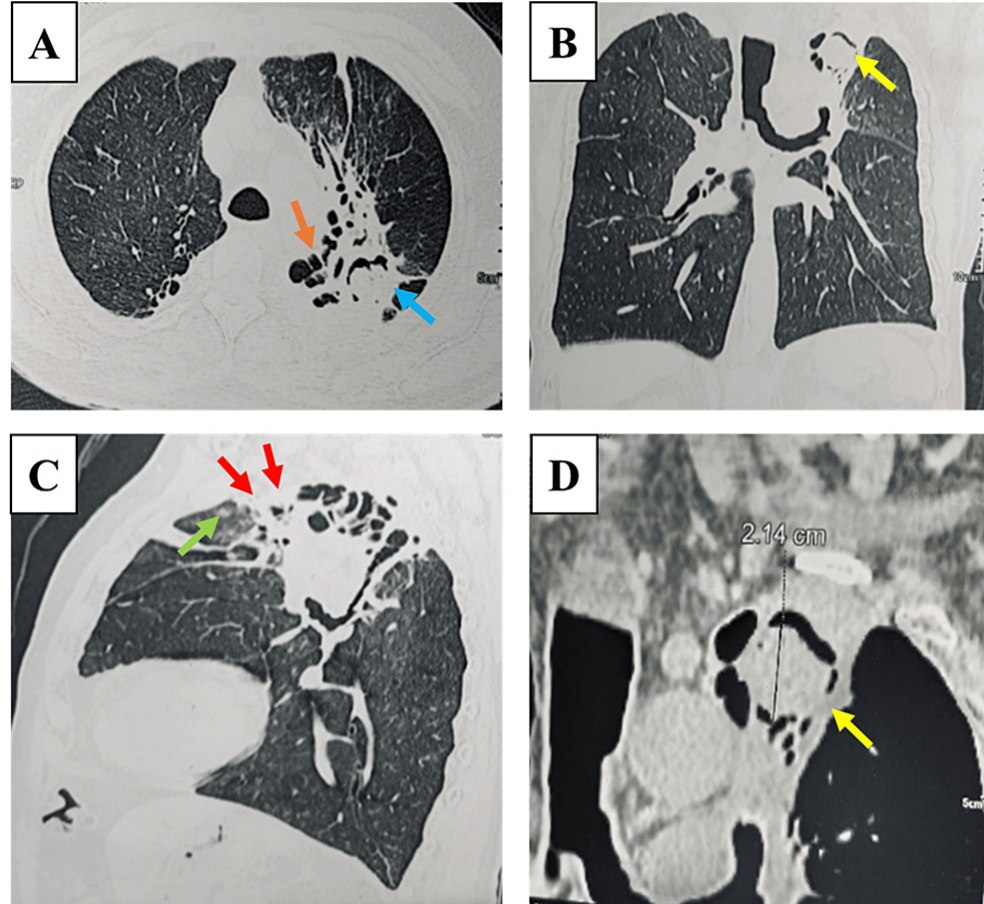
HRCT chest findings A) Lung window in axial section shows left upper lobe fibro-cavity with intracavitary mass (blue arrow) with shaggy cavitary wall and bronchiectasis in atelectatic segments (orange arrow). B) Lung window in coronal section shows air crescent sign (yellow arrow) and bilateral diffuse ground glass opacities. C) Sagittal section shows parenchymal infiltrates (green arrow) and pleural thickening adjacent to the cavity (red arrow). D) Mediastinal window reveals mixed attenuating soft tissue density with an air crescent sign in the left upper lobe (yellow arrow).
